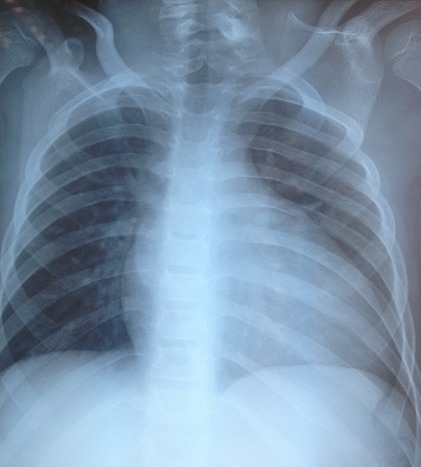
Radiographie du thorax face montrant la surélévation de l’épaule gauche
